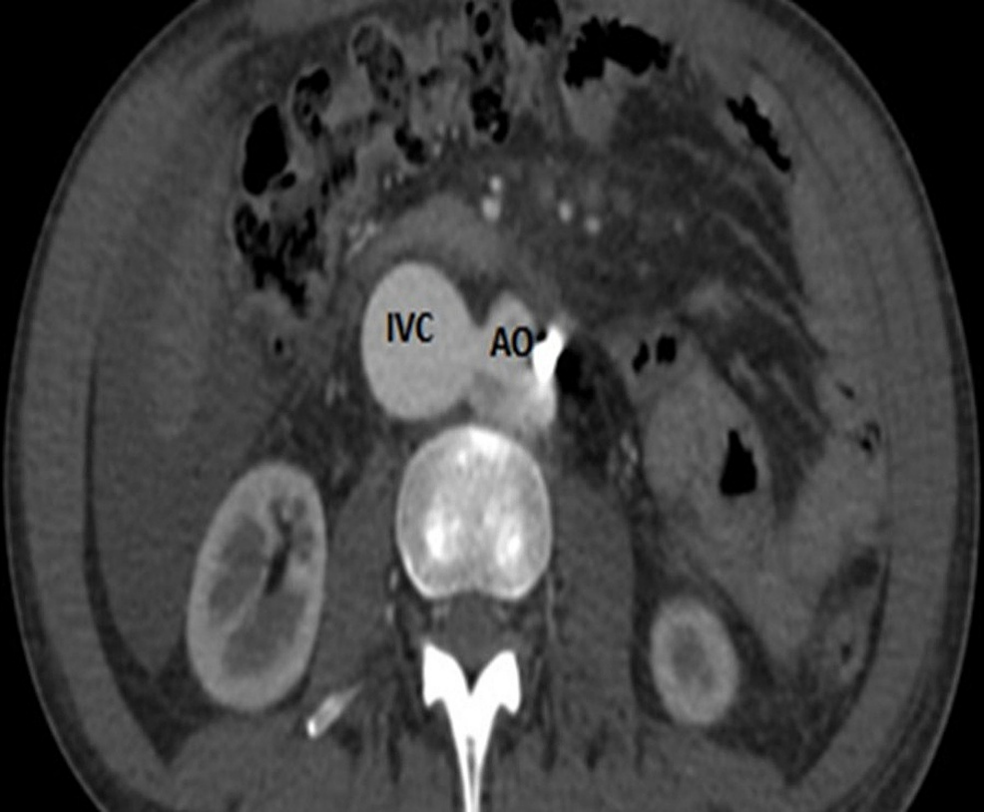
Computed tomography angiography (Axial view) Shows the communication between the aorta (AO) and the inferior vena cava (IVC) during the arterial phase. we also note the presence of the foreign material in the AO.
